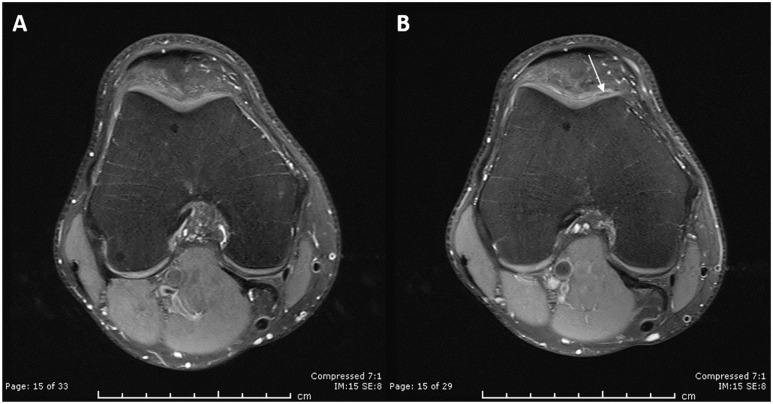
MRI analysis of a healed chondral harvest site. Representative protein density with fat saturation (PD-FS) MRI images taken
